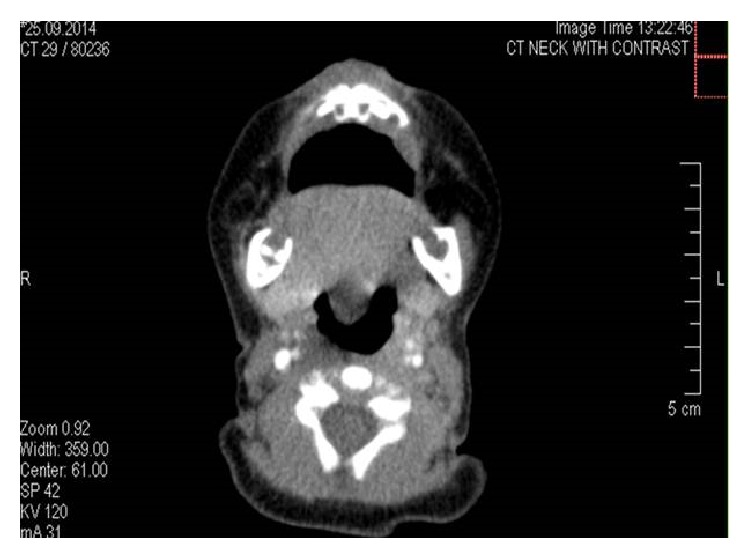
Axial CT scan showing a retroglossal fairly defined rounded nonenhancing hypodense cystic lesion measuring about 9 mm, seen projecting into oropharynx.
